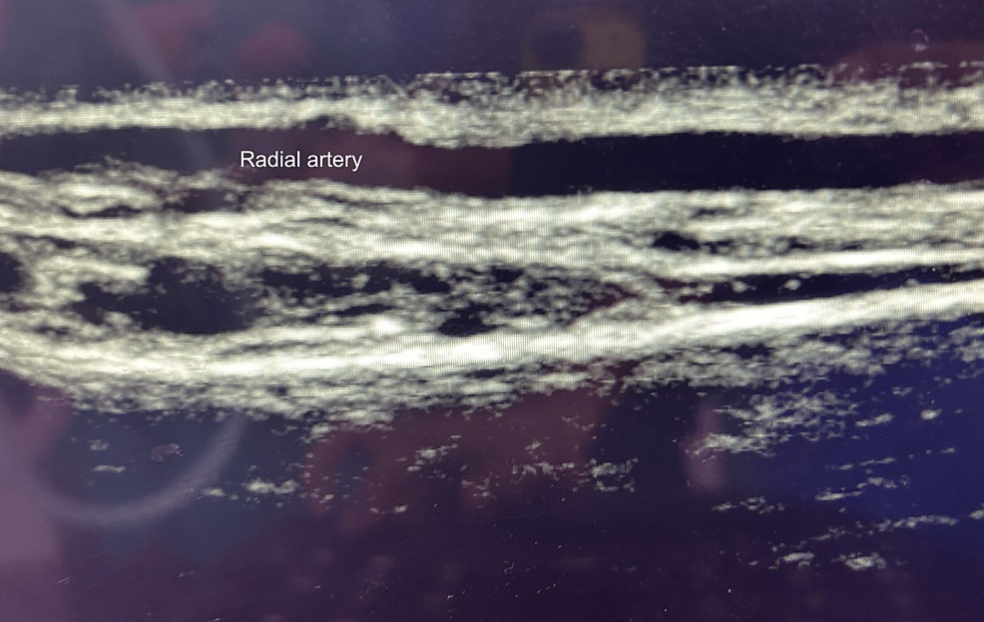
Ultrasound view of radial artery (longitudinal section)
